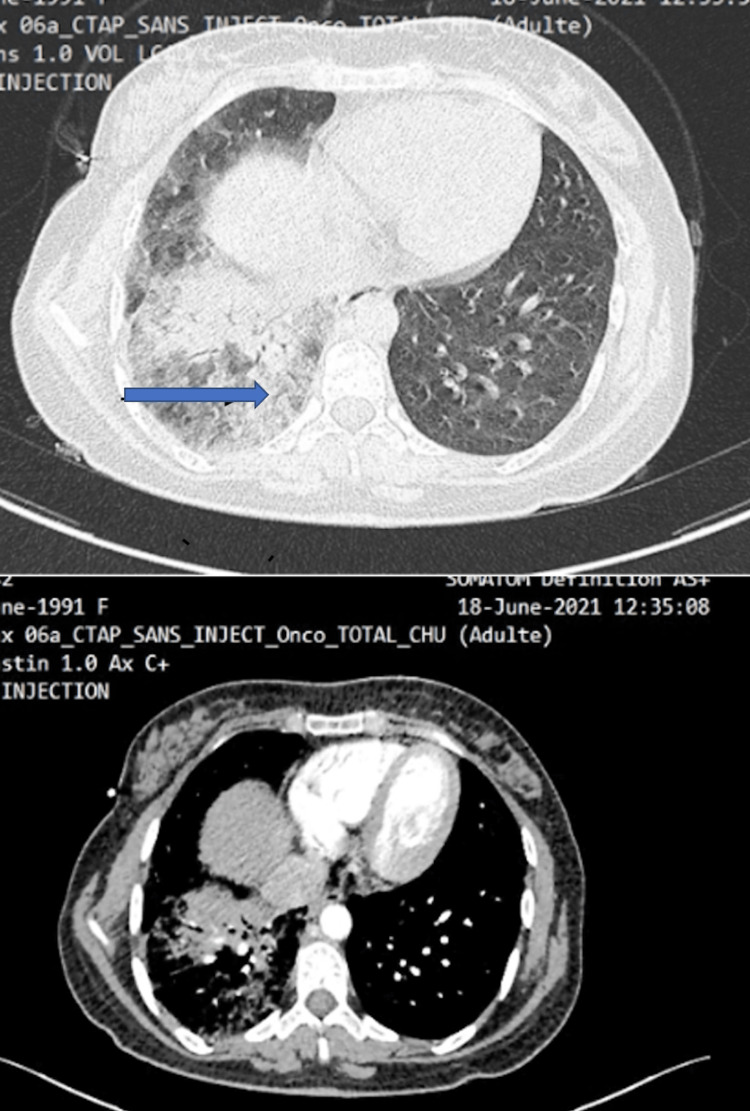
Thoracic CT scan revealed a focus of alveolar consolidation in the right lower lobe (blue arrow).
